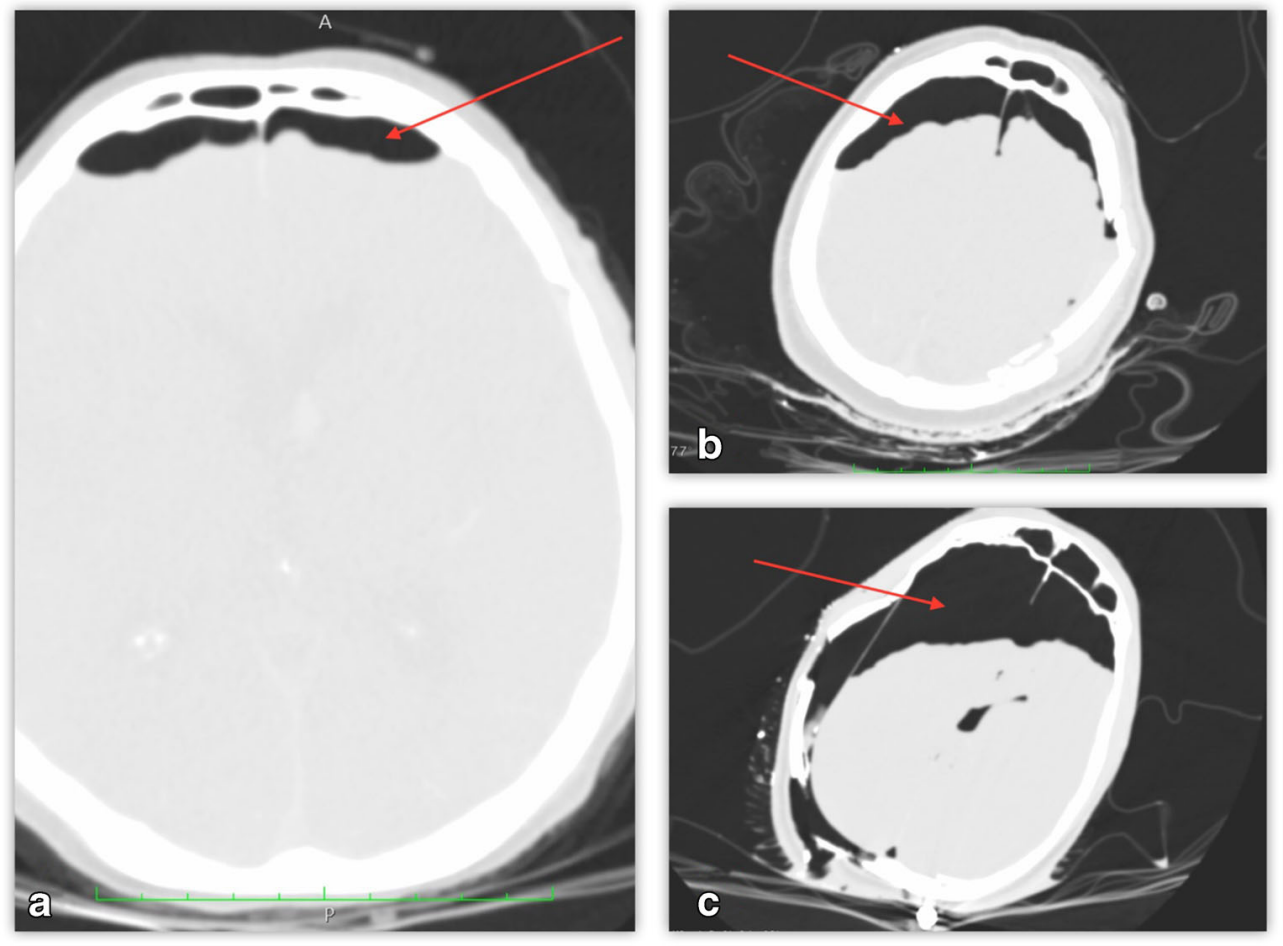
Postmortem computed tomography (PMCT) multiplanar reconstruction (MPR) images showing semi-quantitative analysis of pneumocephalus (PNC). a PNC I, b PNC II, c PNC III
